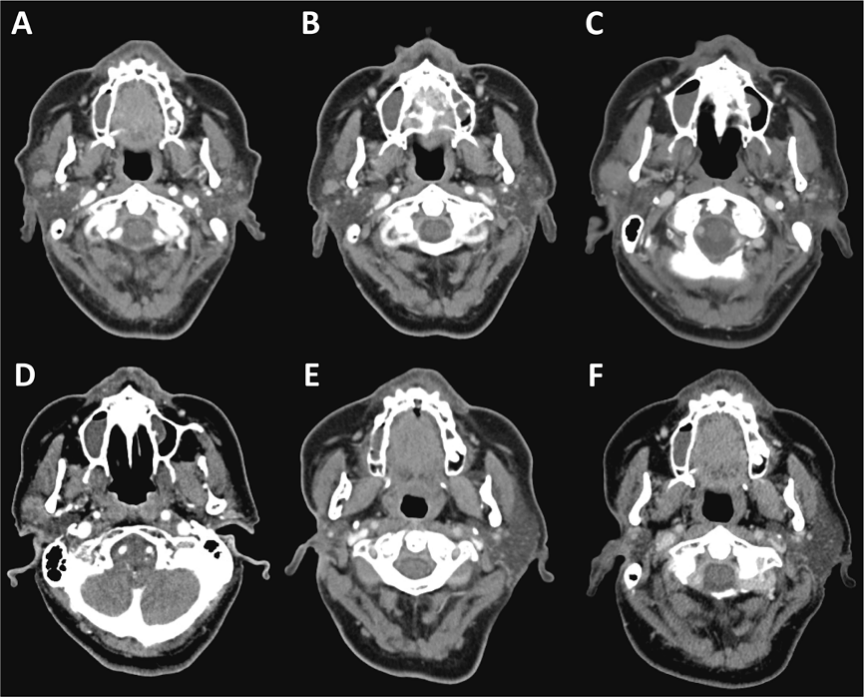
Computed Tomography (CT). A CT showing solid lesion in right parotid gland (Baseline), B Stable Disease under tamoxifen treatment. C Growth of the solid lesion in right parotid, D Stable Disease after switch to anastrazol, E Postsurgical CT showing absence of right parotid gland F Stable disease in surveillance.
